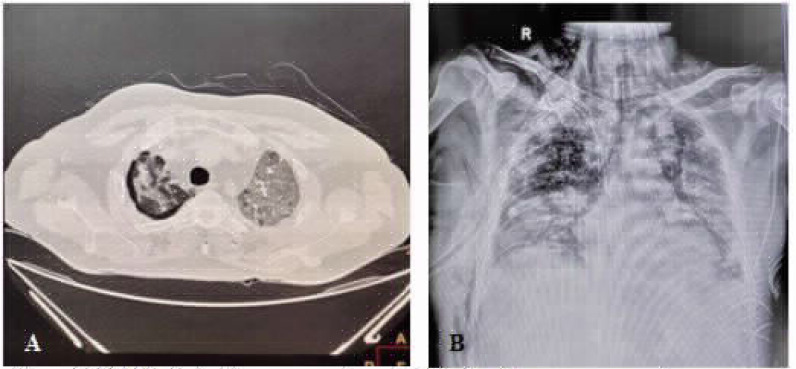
CASE 6: A: right pneumothorax in CT, B: subcutaneous emphysema with drain in situ
